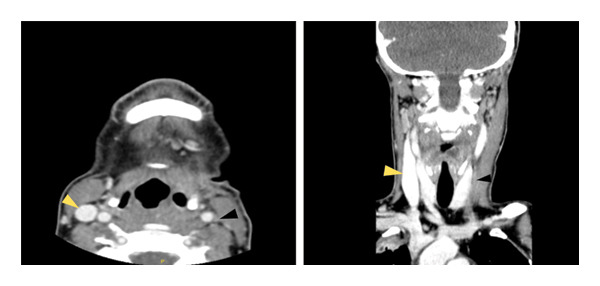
CT‐scan (axial and coronal view) where a left thread‐like internal jugular vein (black arrows) can be seen. The right jugular vein is as well marked (yellow arrows).
